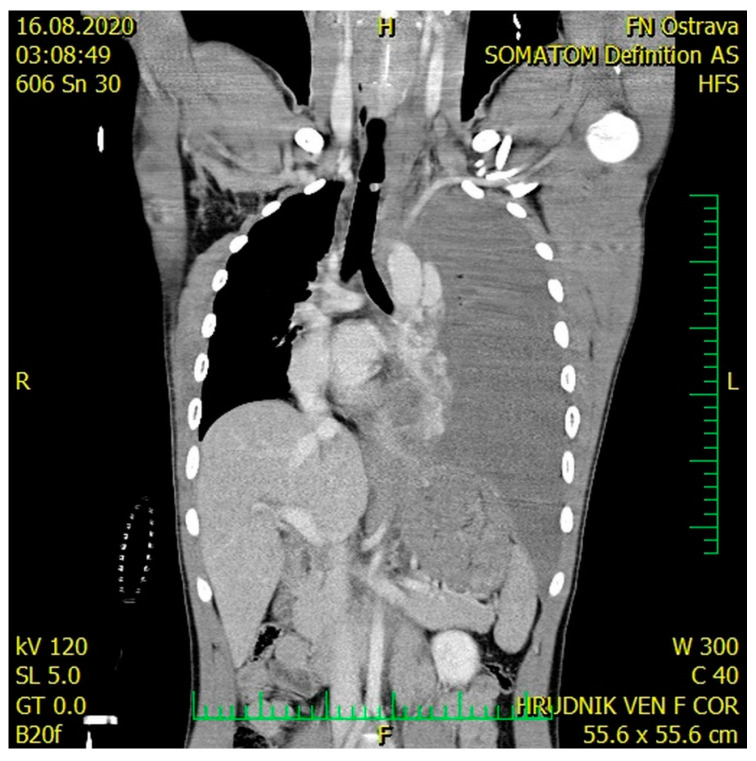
CT scan—large left-sided tension hemothorax with the mediastinal shift.
